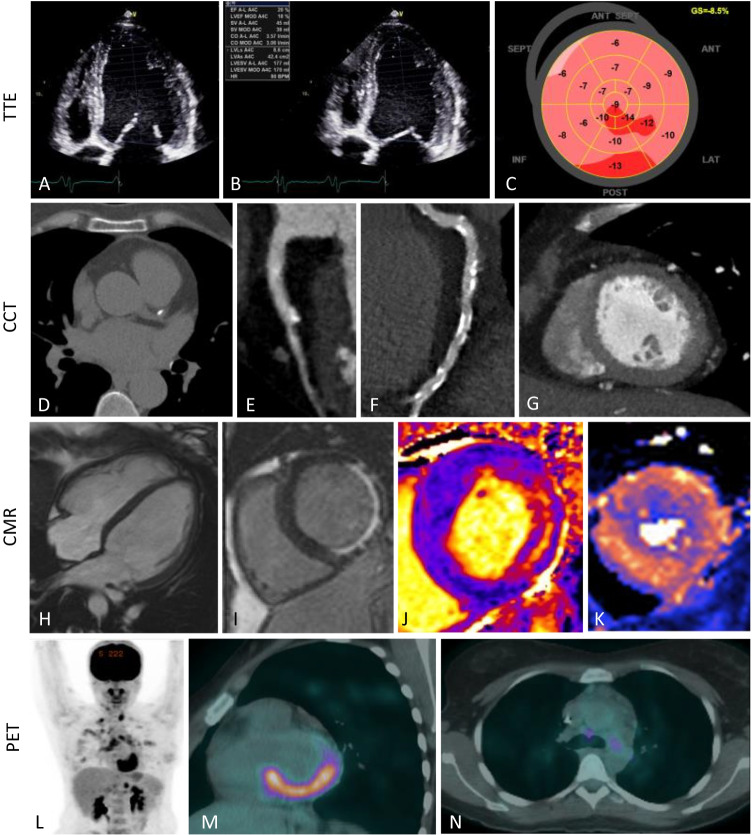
Varying imaging modalities and causes of heart failure in women. TTE, (transthoracic echocardiogram) — images A–C. Patient with non-ischaemic cardiomyopathy. Apical 4-chamber view in diastole (A) and systole (B) showing severely impaired systolic function (LVEF 20%) by Simpson’s Biplane. Strain map showing globally reduced longitudinal strain (C). CCT, cardiovascular computed tomography — images D–G. Coronary artery calcium scoring (D), curved reformats showing mixed calcified and non-calcified atheroma (E) and calcified plaque (F), and short axis cine (G). CMR, cardiovascular magnetic resonance — images H–K. 4ch cine of dilated heart (H), subendocardial infarct on LGE images (I), mid-wall inflammation of myocarditis seen on T1 map (J), global subendocardial perfusion defect on short axis perfusion map (K). PET, positron emission tomography — images L–N. Focal intense FDG uptake in the left ventricle (D–E) and affecting the basal inferoseptum, inferior, inferolateral, and lateral walls (E). Axial slice showing FDG avidity in the mediastinal lymph nodes (F) in a patient with a history of systemic and cardiac sarcoidosis
